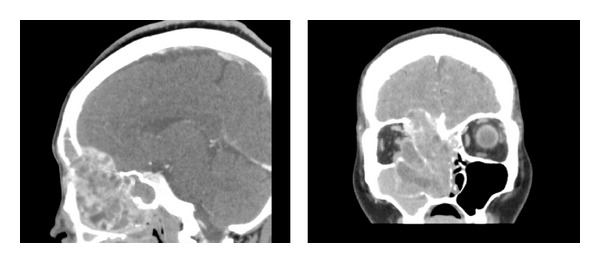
Preoperative computed tomography (contrast-enhanced) demonstrates the mass occupied right nasal cavity with intracranial invasion and extension into the orbit.
